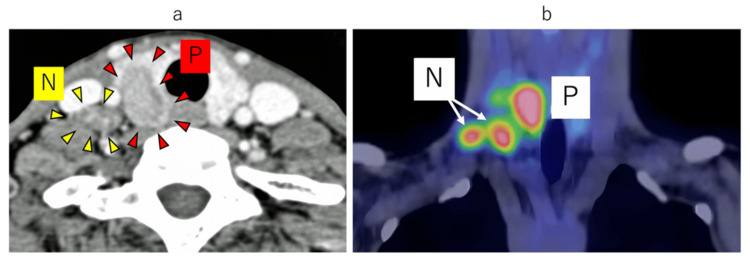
Contrast-enhanced CT axial (a) and PET-CT coronal (b) N: lymph node; P: primary tumor; CT: computed tomography; PET: positron emission tomography
